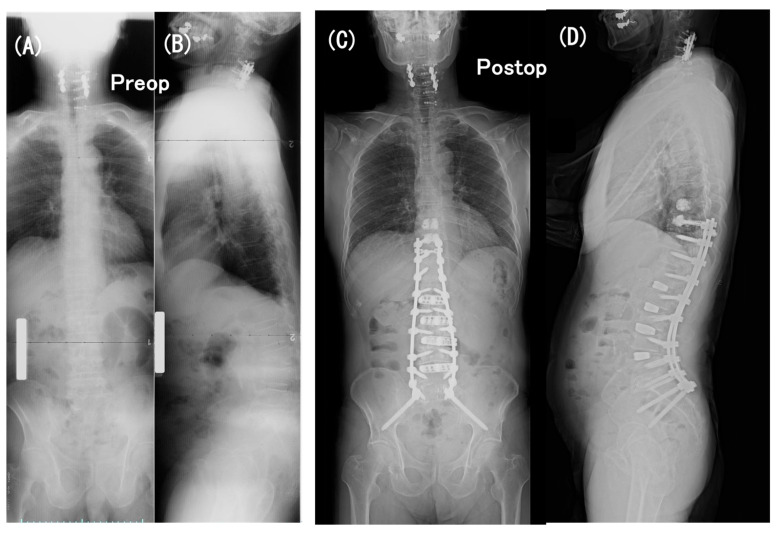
A 73-year-old man with persistent low-back pain and loss of ambulatory endurance with severe sagittal spinopelvic imbalance. Coronal (A) and sagittal (B) full-length standing radiographs obtained preoperatively revealed the following: LL = −19°, PI = 40°, PI–LL = 59°, PT = 38°, TK = 5°, and SVA = 118 mm. Coronal (C) and sagittal (D) full-length standing radiographs obtained at an early postoperative period. A two-stage operation, one week apart, comprised (1) the first-stage operation for LLIF procedure at L1-2 through L4-5 with the patient in the lateral position and (2) the second-stage operation for posterior instrumentation with the patient placed prone, consisting of PPS fixation at L1 through S1, mini-open transforaminal lumbar interbody fusion (TLIF) at L5-S1 with S2-alar-iliac screw fixation, and a traditional open approach for PS fixation and fusion at T10 through T12 with prophylactic PMMA cement augmentation at T9 and T10. Postoperatively, sagittal spinopelvic parameters improved to 47° in LL, to −6° in PI–LL, to 9° in PT, to 35° in TK, and to −8 mm in SVA.
